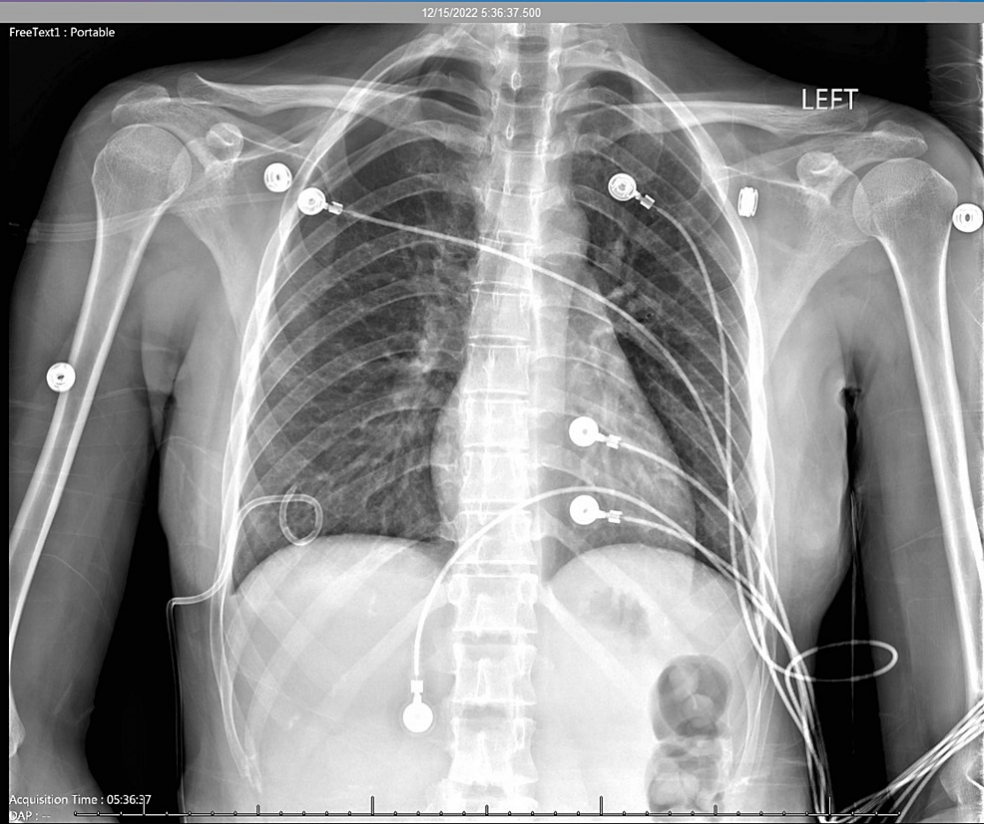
Chest x-ray #4 Impression: No new significant focal consolidation identified with continued interval improvement of right pneumothorax, now measuring approximately 15%-20%.
